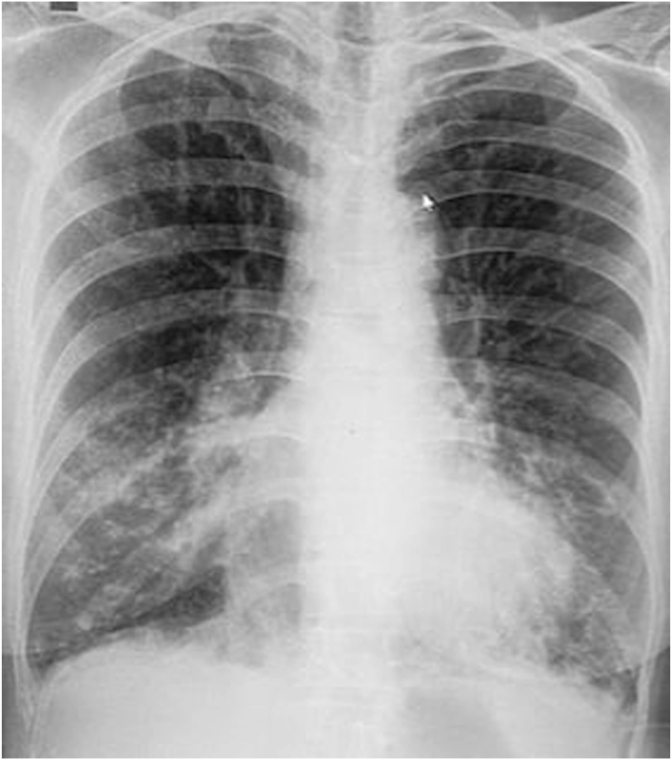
Plain chest X-ray showed bilateral consolidation in the basal lung.
